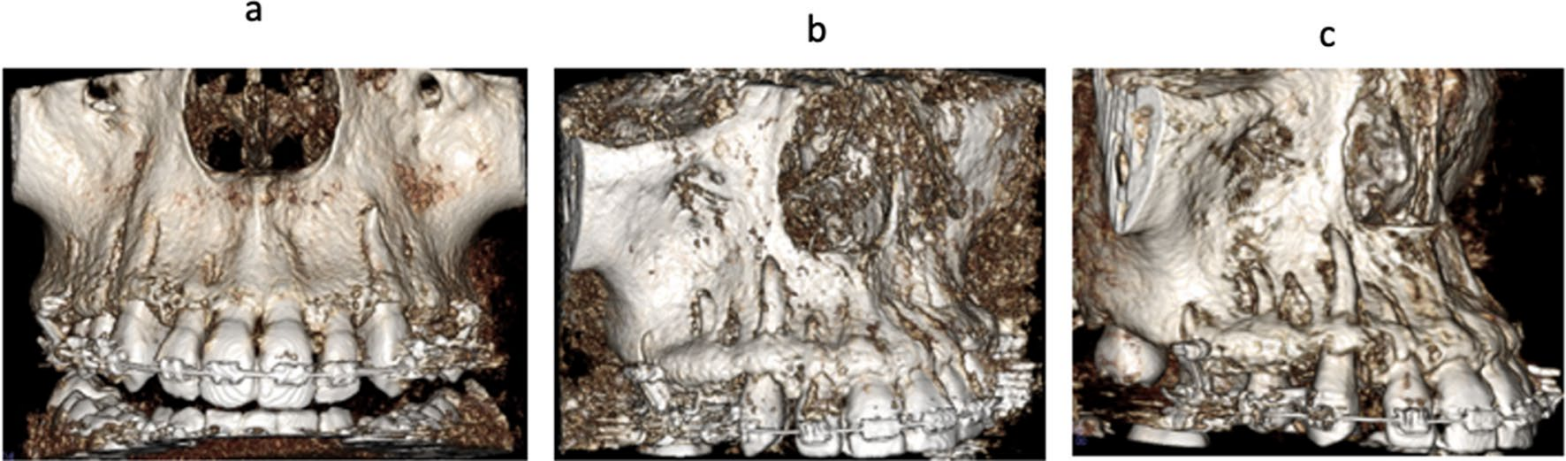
CBCT volumes examined in the 3D solid bone view.
